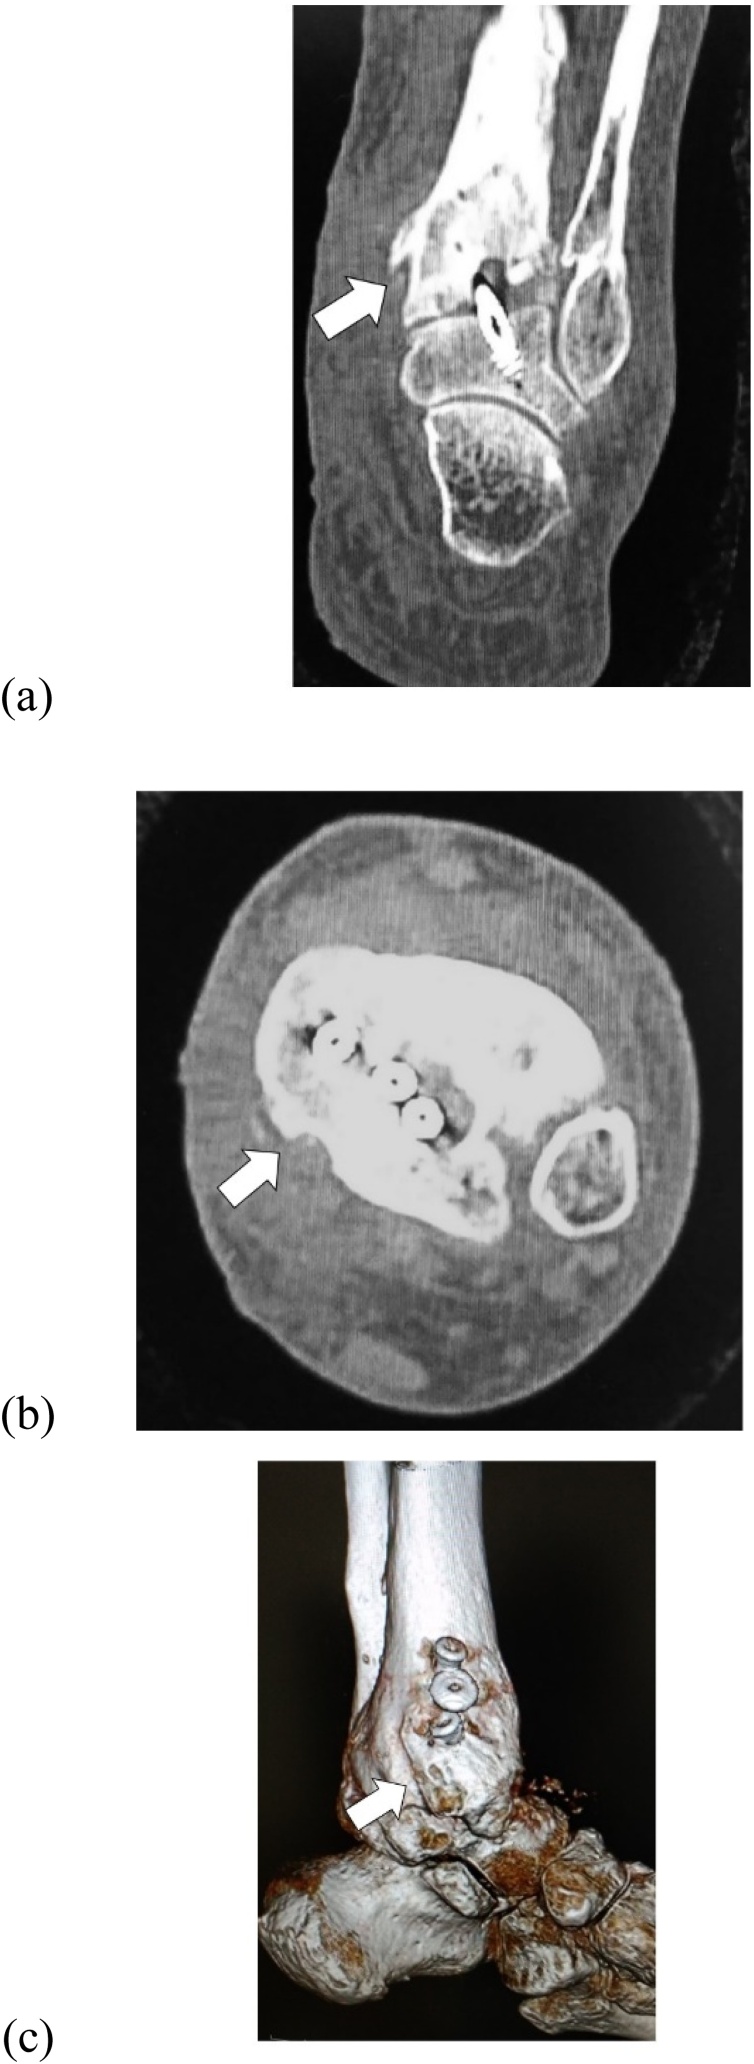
Postoperative computed tomography (CT) images. Osteophyte removal (arrow) on (a) axial, (b) coronal, and (c) 3-DCT images.
